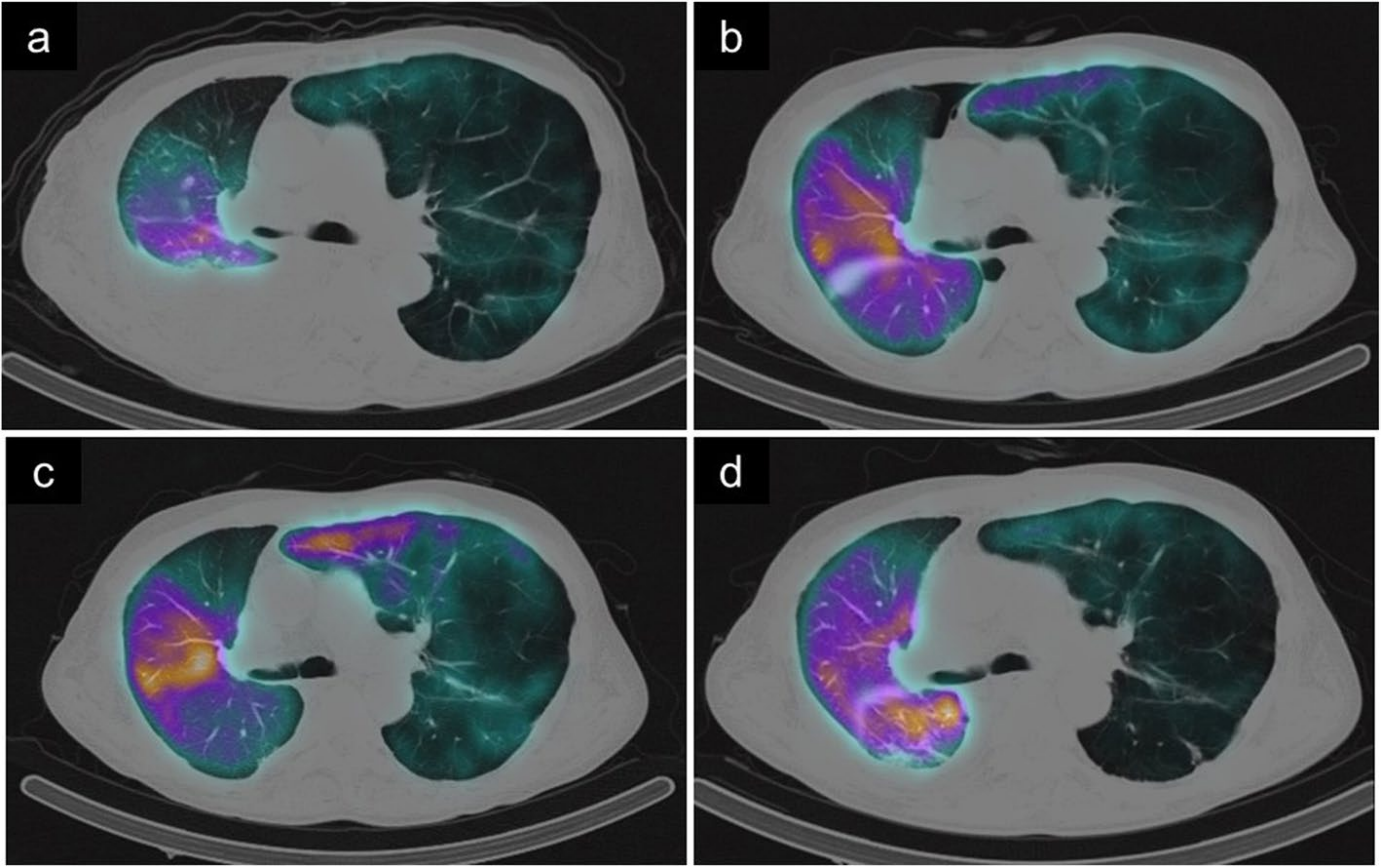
Pulmonary perfusion scintigraphy after right lung transplantation and pulmonary artery plasty. The pulmonary right-to-left blood flow ratio by pulmonary perfusion scintigraphy was 37:63 at 2 weeks after transplantation (a) and 49:51 at 7 weeks after transplantation (b). However, at 10 weeks, the flow to the transplanted lung decreased, showing a ratio of 43:57 (c). At 5 weeks after pulmonary artery plasty, pulmonary perfusion scintigraphy showed an increased flow to the right lung, with a right-to-left ratio of 67:33 (d)
